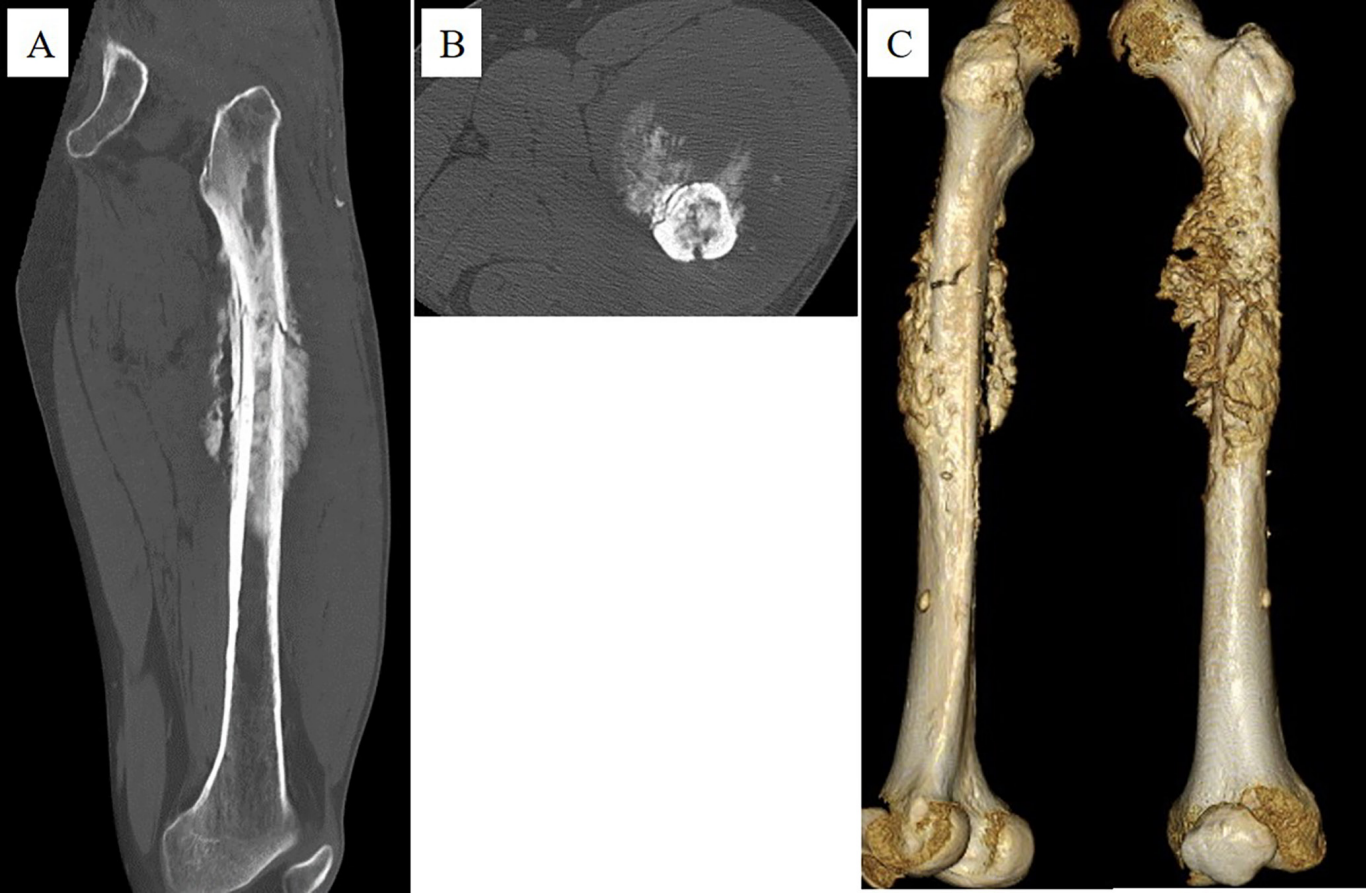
Computed tomography showing extra‐osseous tumor extension with ossifying tumor‐induced calcifications at 8 years after radiation. (A) Sagittal sequence. (B) Axial sequence. (C) Three‐dimensional CT.
